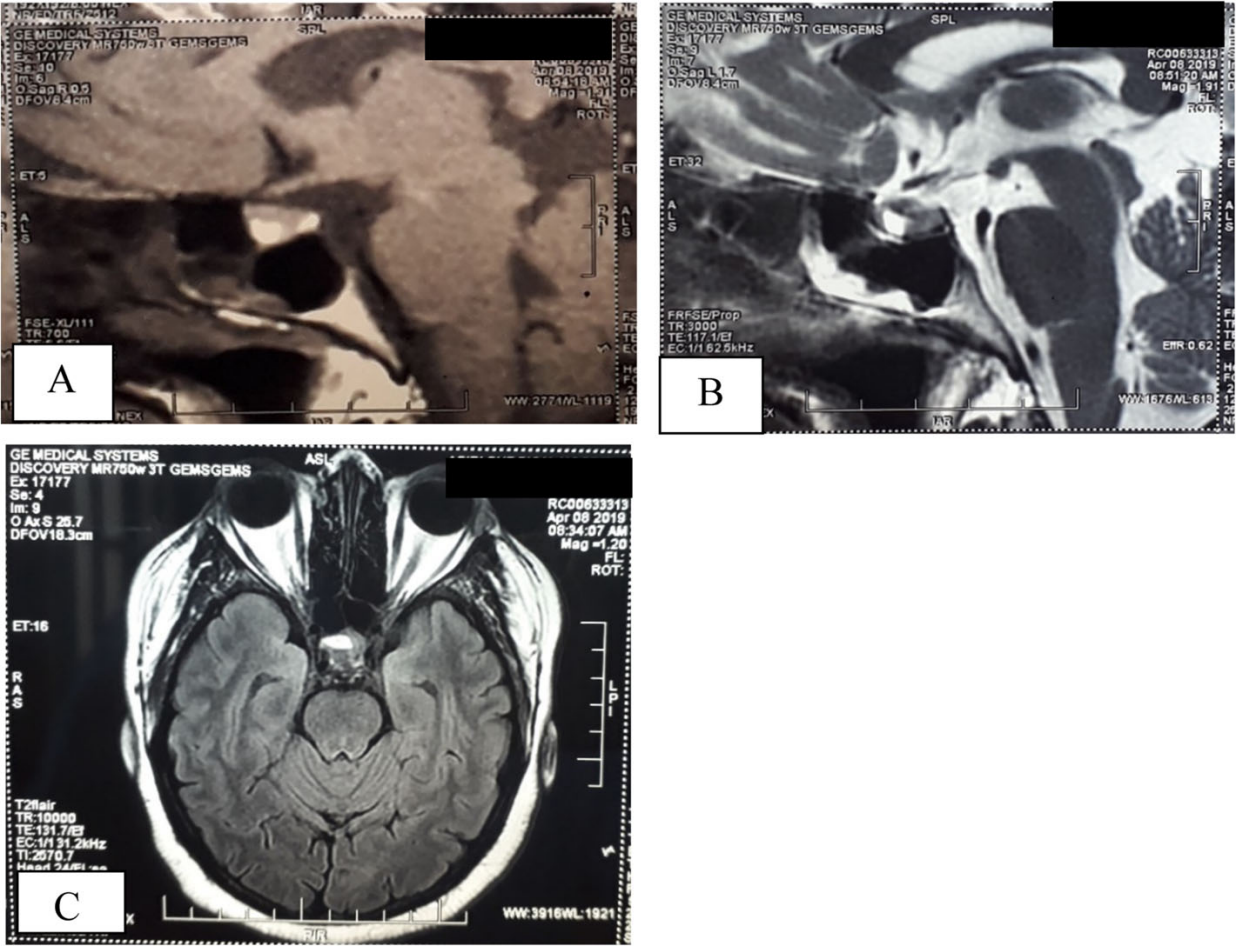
MRI showing high signal lesion of 10x8x9 mm suggestive of pituitary microadenoma. a T1 contrast sagittal. b T2 sagittal. c FLAIR axial
